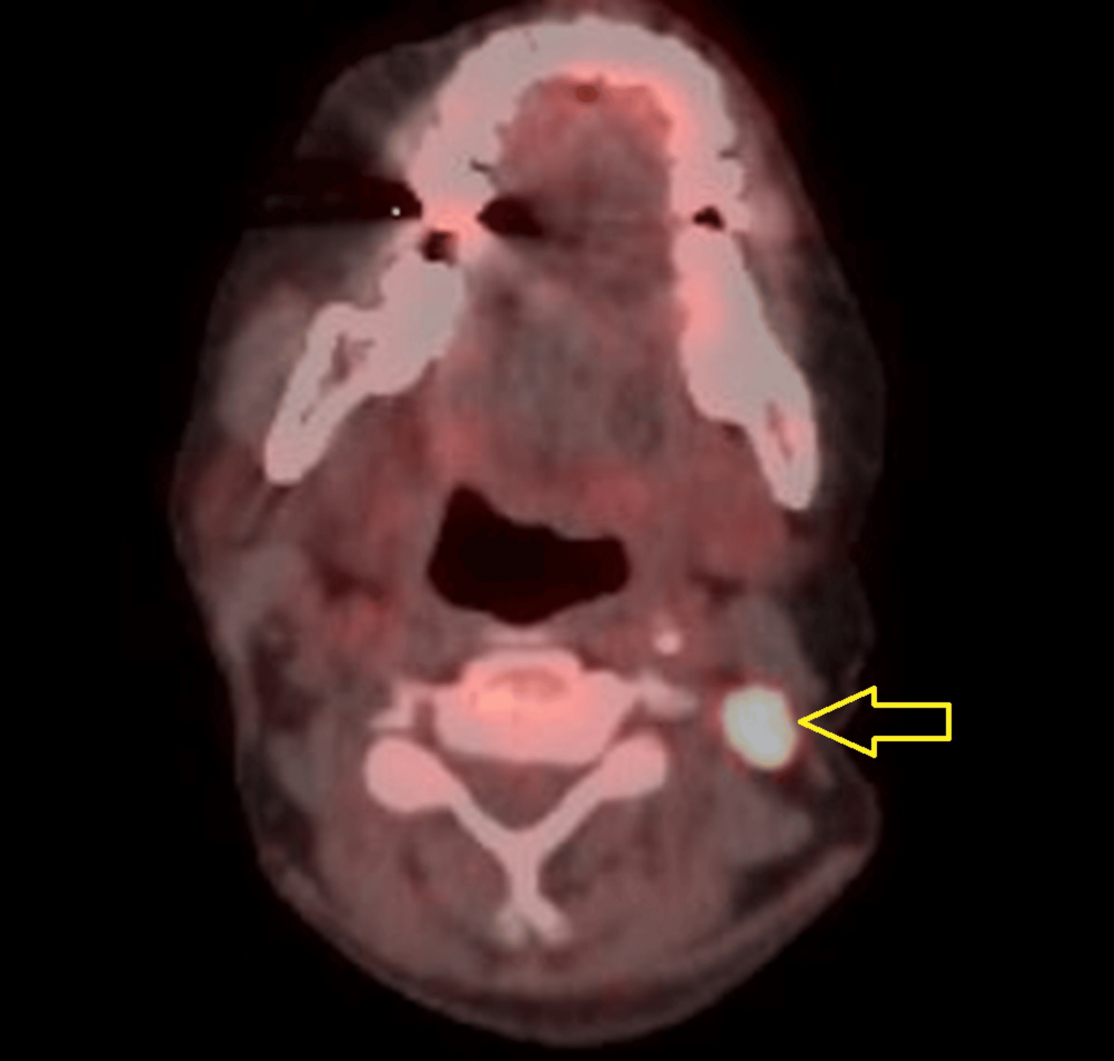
Fluorodeoxyglucose positron emission tomography (FDG PET) scan before treatment Fused axial PET scan demonstrating increased FDG uptake in the left cervical lymph node prior to treatment initiation (arrow).
